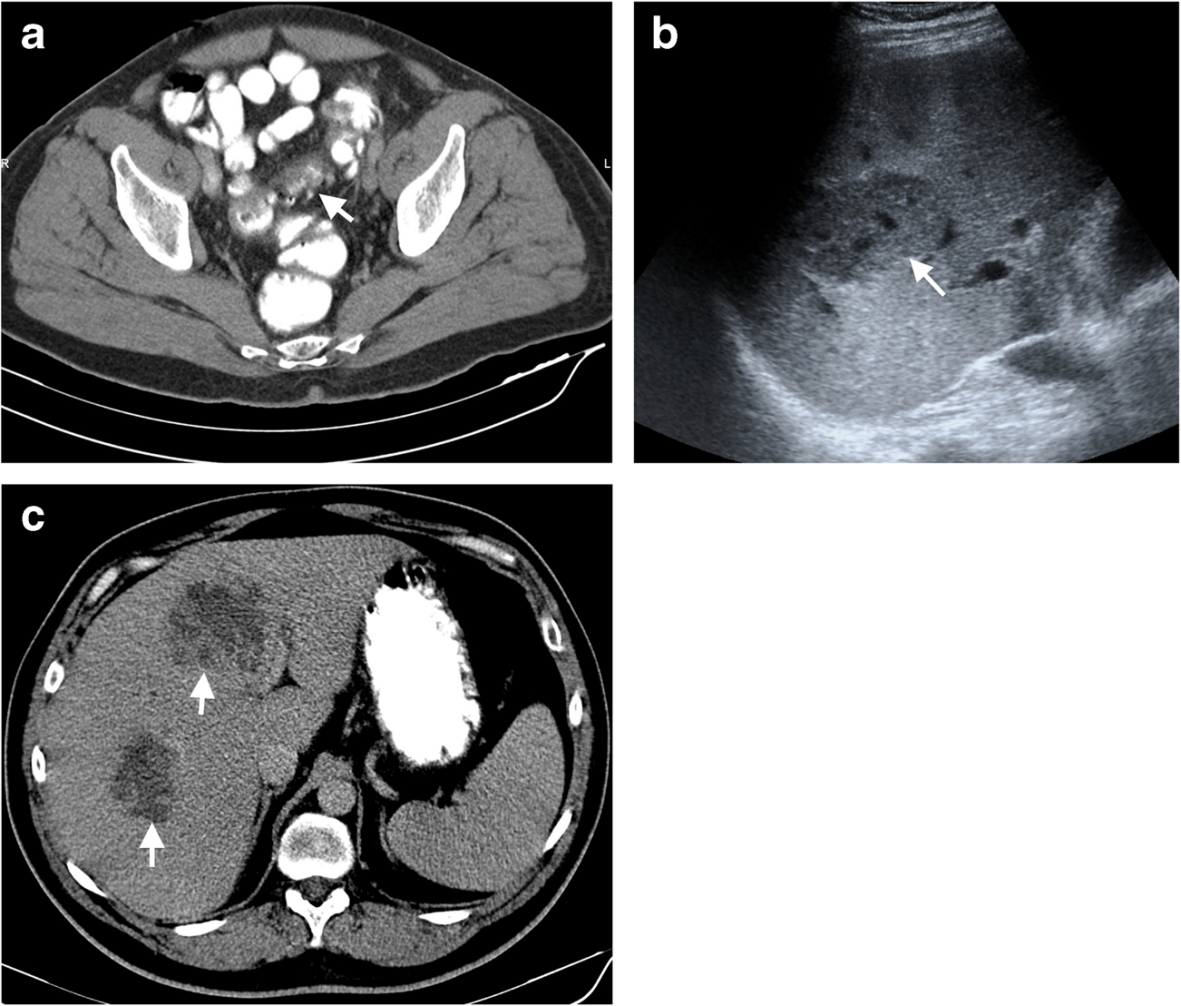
Liver abscess in a 48-year-old male after diverticulitis. a Axial CT demonstrates diverticulitis (*arrow*) in the sigmoid colon. b Liver abscess secondary to diverticulitis. Gray-scale US demonstrates a liver abscess with a hypoechoic, solid mass appearance (*arrow*) in the liver parenchyma. c Liver abscesses manifest with low-attenuating, ill-defined appearance (*arrows*) in the liver parenchyma on axial contrast-enhanced CT
